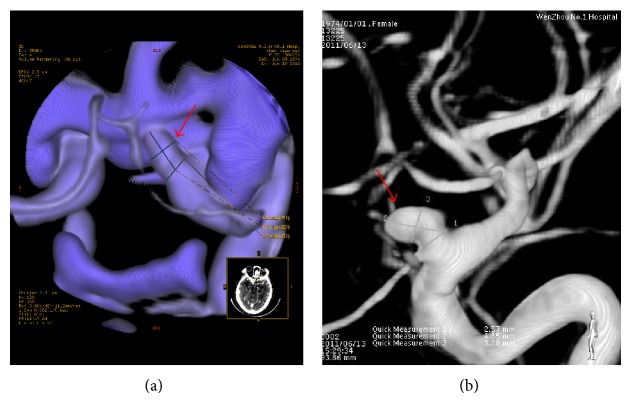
Images from a 37-year-old woman with SAH. The L-CTA (a) and 3D-DSA (b) showed the same aneurysm at the same location. The maximum diameter of aneurysm and neck sizes in C-CTA (a) and 3D-DSA (b) were 4.5, 2.7 mm and 4.85, 2.57 mm, respectively (red arrow).
